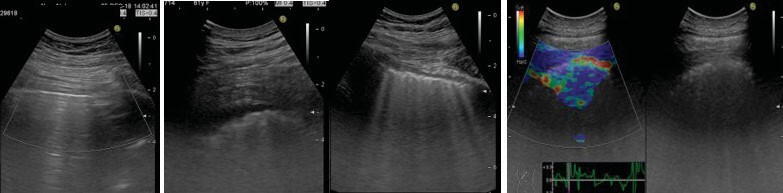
Figure 1. Samples from lung ultrasonograpghy
